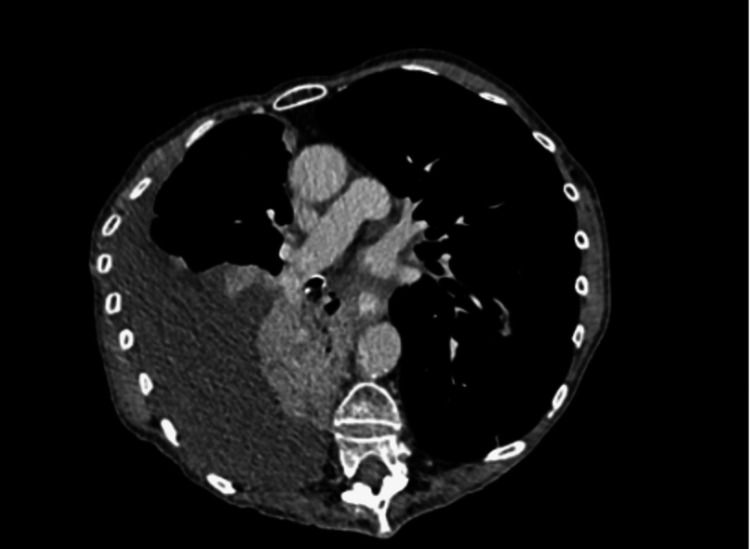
A CT scan of the chest shows a right-sided pleural effusion with a collapse of the right lower lobe.
